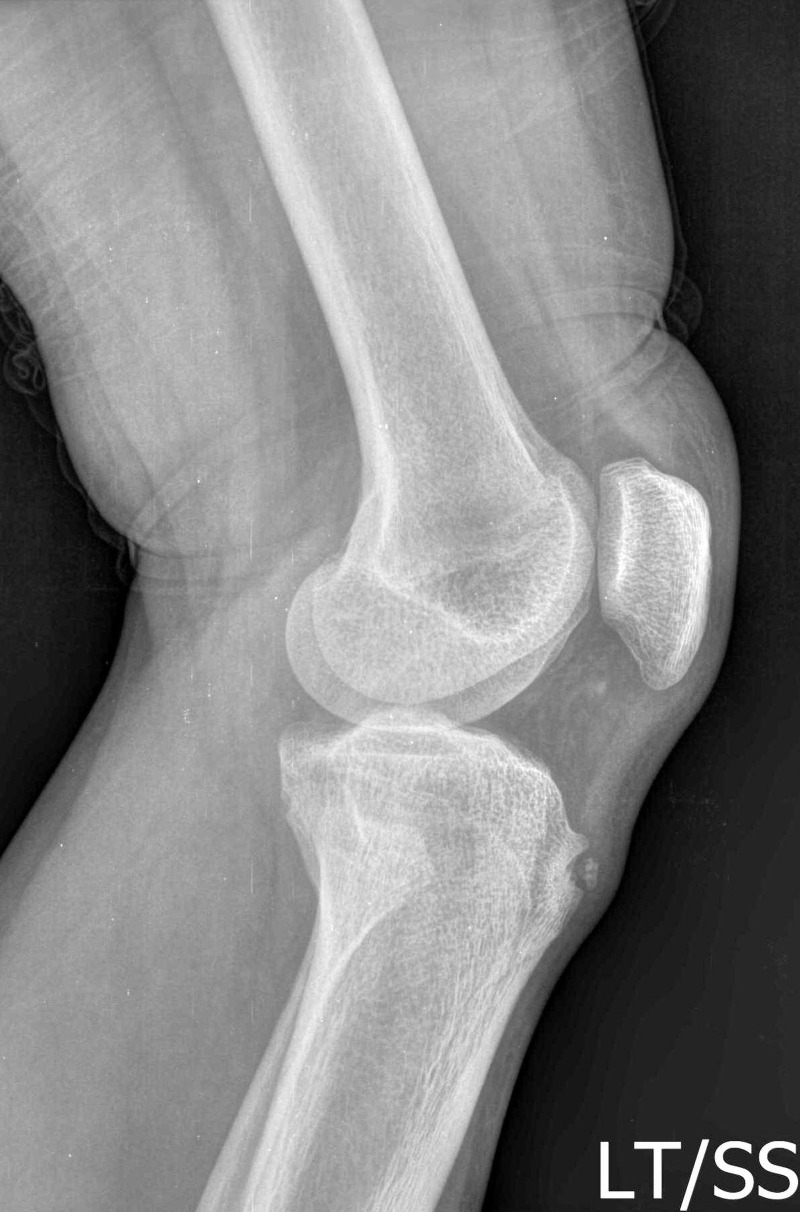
Separated ossicle and bony prominence seen in lateral radiography of knee in OSD.
